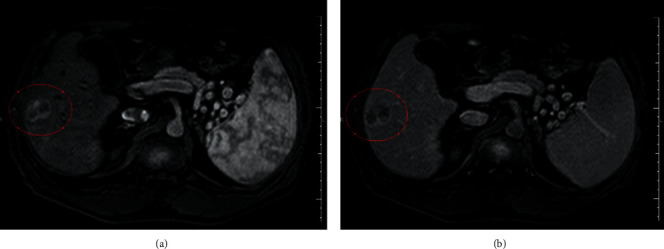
Magnetic resonance imaging performed on March 31, 2017, showing the initial liver tumor that measured 3.0 × 2.5 cm in liver segment VI with arterial enhancement and a marked washout in the portal phase (the tumor is underscored by the red line). (a) Arterial phase. (b) Portal phase.
